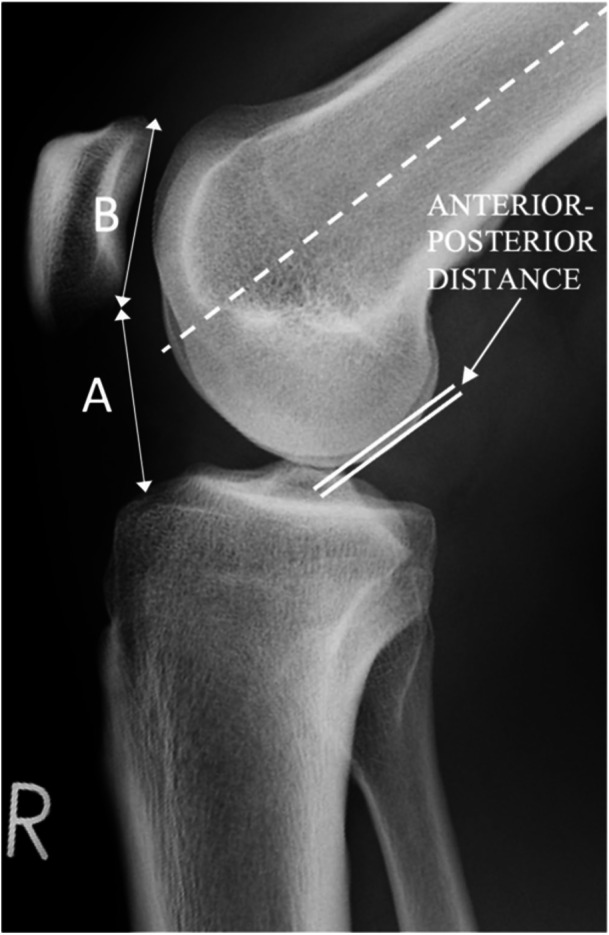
Measurement of CDI and APD. Lateral radiograph of a right knee showcasing measurements of patellar height using the CDI (A/B), and measurement of the APD. Radiograph is cropped for visualisation. APD, anterior–posterior distance; CDI, Caton‐Deschamps Index.
